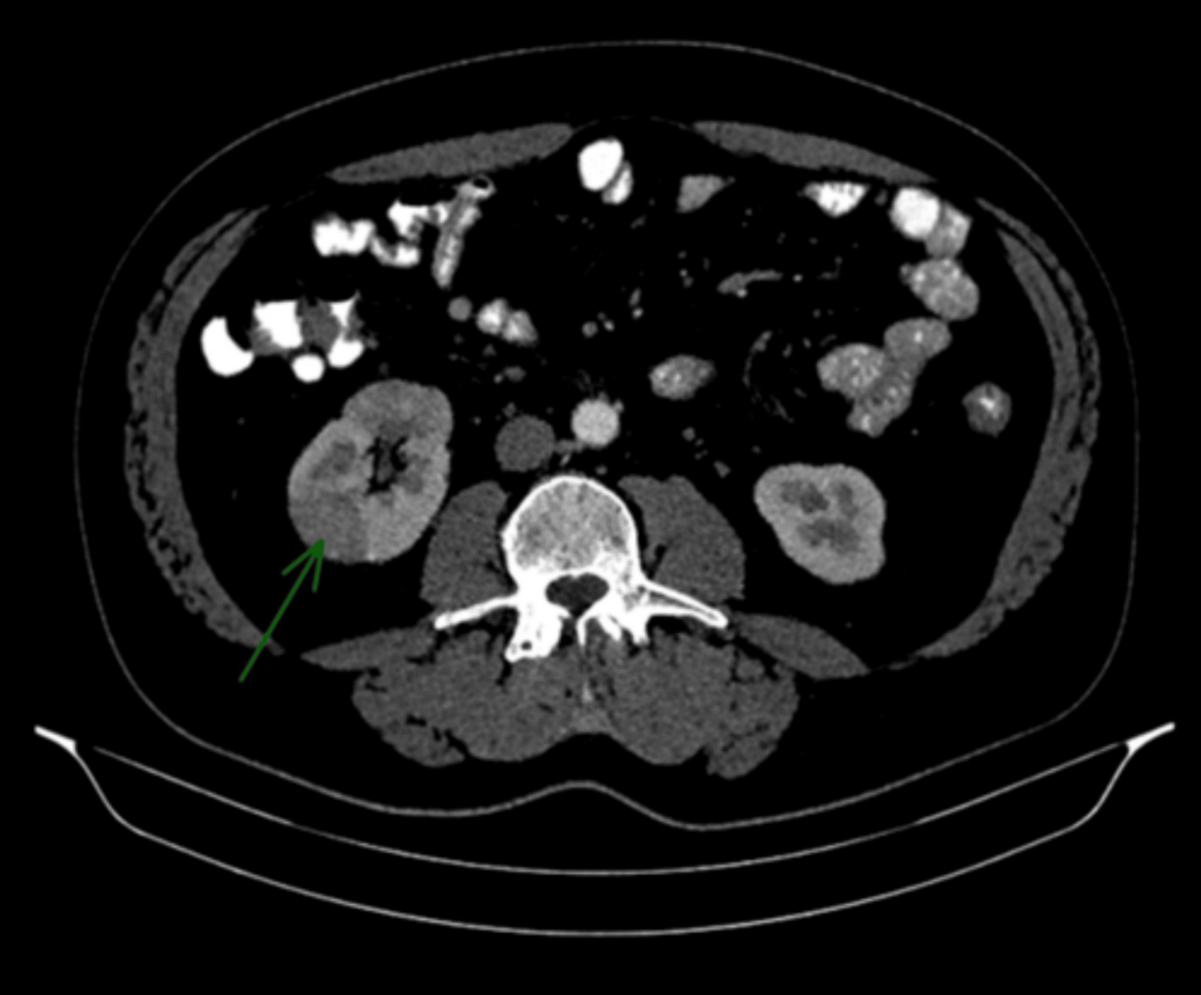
Contrast-enhanced CT of the abdomen (axial view) Right-sided focal nephronia, characterized by ill-defined, low-attenuation cortical changes without abscess formation
